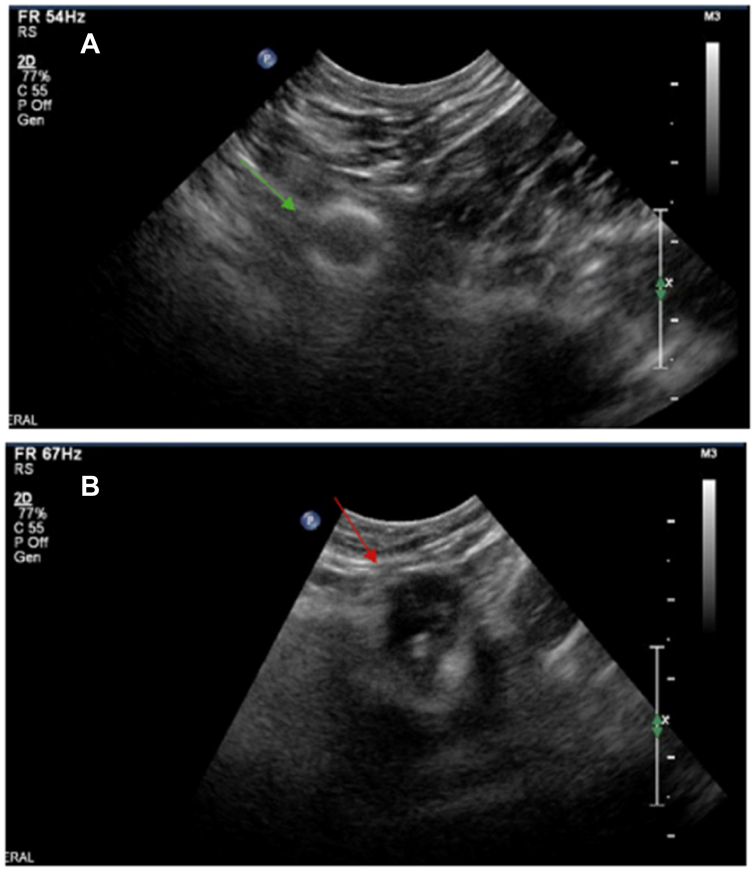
Postoperative duplex ultrasound image showing (A) stable placement of the vascular plug within the chest wall varix (*green arrow*) and (B) thrombosed, noncompressible chest wall varix (*red arrow*).
